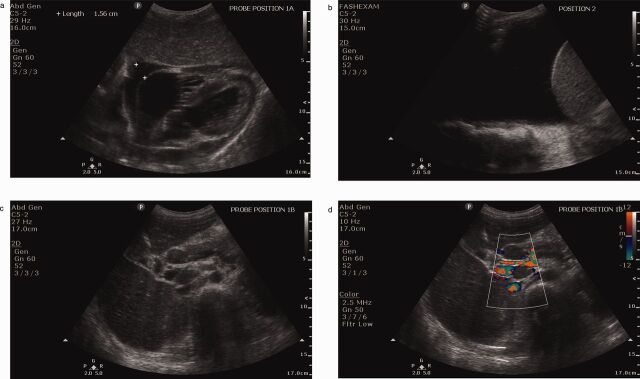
Images of Findings From Focused Assessment With Sonography of HIV-Associated Tuberculosis Protocol in Participants With Signs and Symptoms of Tuberculosis (a) Anechoic fluid surrounding the heart consistent with a moderate pericardial effusion. (b) Anechoic area superior (left) to the diaphragm and liver consistent with a large right pleural effusion. (c) Isoechoic nodules consistent with peri-portal and para-aortic lymphadenopathy. (d) Color Doppler further differentiates vasculature from lymph nodes.© 2016 Daniel Kahn/UCLA
